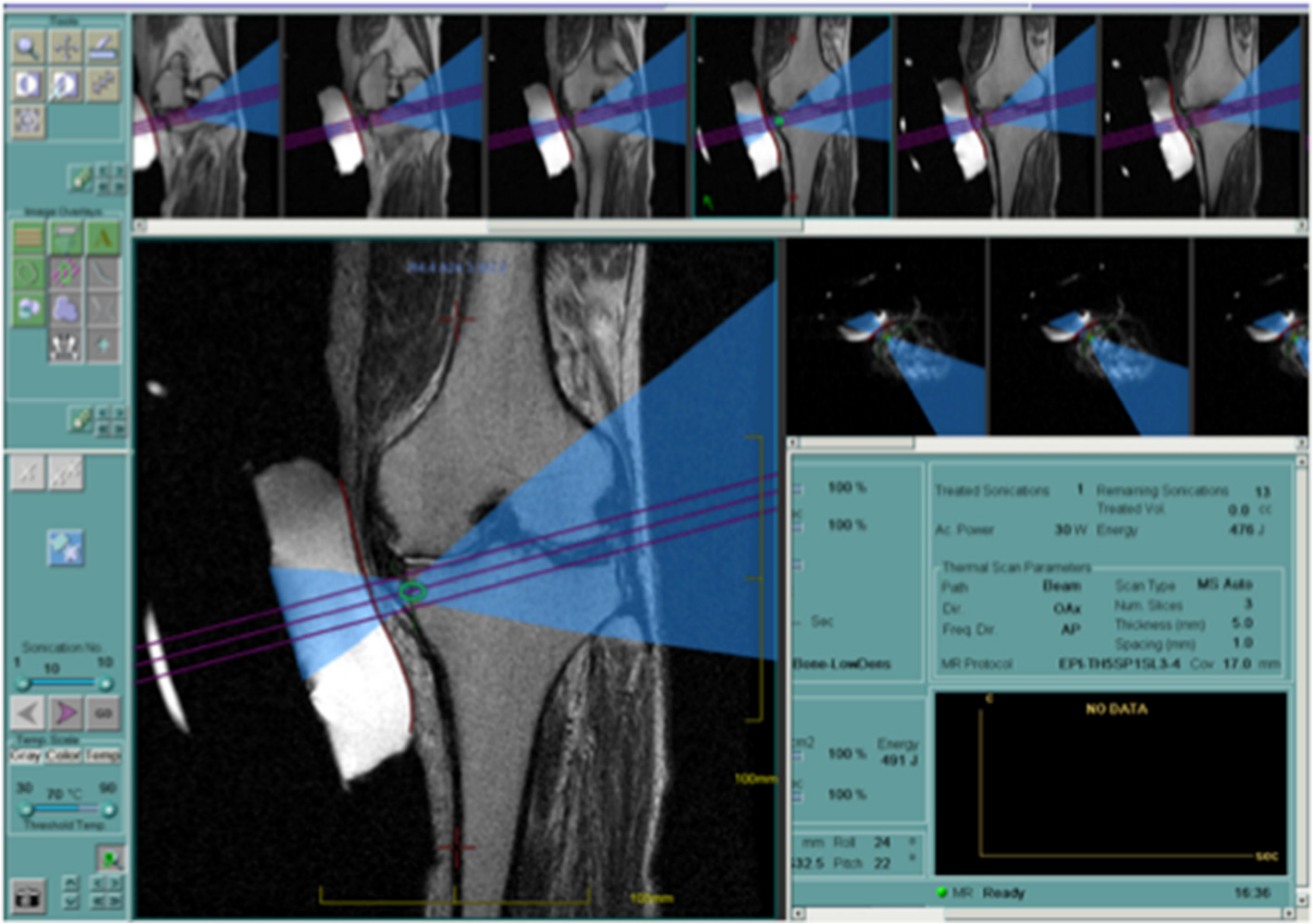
Three-dimensional treatment planning. Sonication site and ultrasound beam pathway are indicated by the examiner. The system automatically generated the optimal treatment plan including energy levels and number of sonications.
